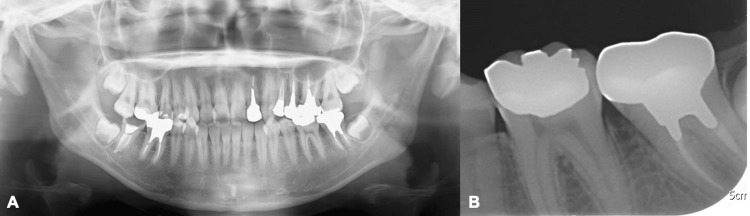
Preoperative X-ray images A. Preoperative panoramic imaging. B. Dental imaging. Alveolar bone resorption is found between the mandibular first and second molars.
